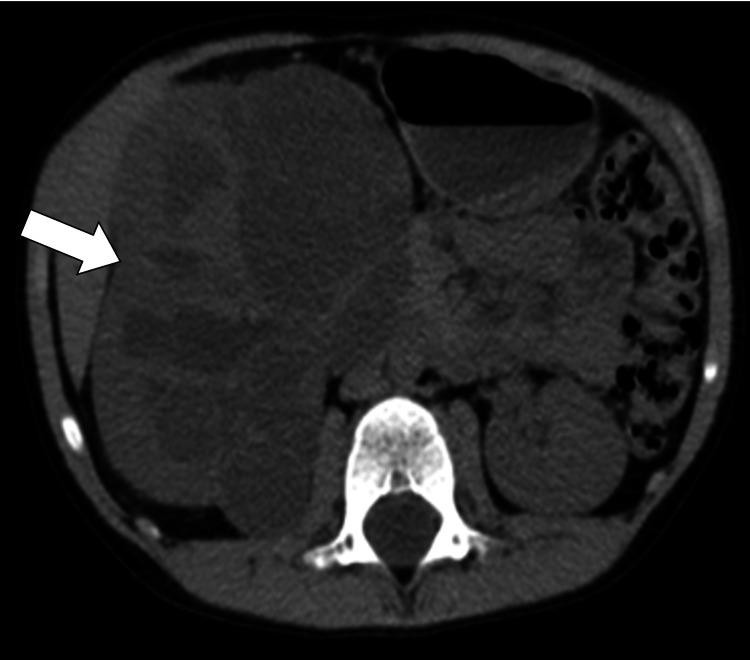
Axial non-enhanced CT scan showing multiloculated well defined soft tissue lesion arising from the right kidney measuring 11x8x7.5 cm (arrow)
